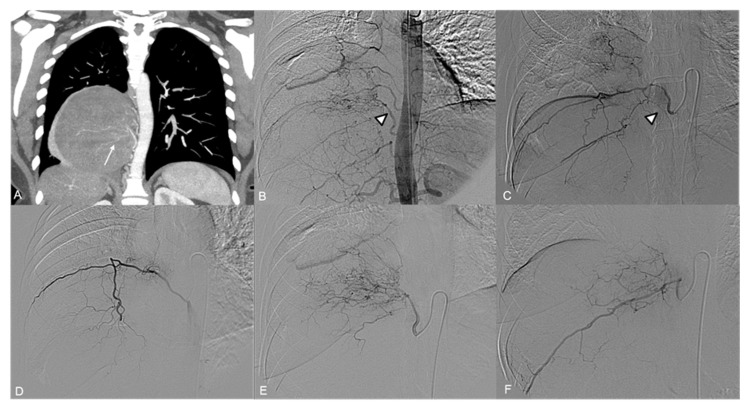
(A) CT angiography, coronal view: large right paravertebral thoracic mass with multiple arterial feeders (arrow). (B) The preliminary aortography demonstrates a rich vascularization of the tumor, mainly by branches from the VI, VIII, and X intercostal artery and an aberrant aortic branch (arrowhead). (C–F) Selective angiography of the principal arterial feeders; note a radiculomedullary artery arising from the VIII intercostal artery (arrowhead in (C)).
